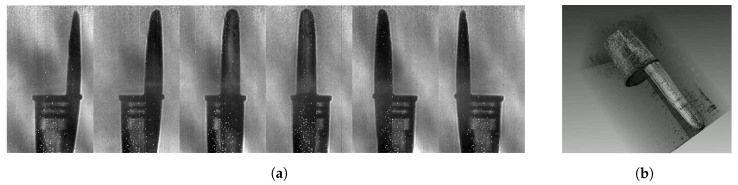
(a) Selection of 6 cropped and centered 2D projections of the sample among the 36 acquired from 0° to 175° (b) 3D isometric visualization of the pen cap reconstructed by terahertz computed tomography.
